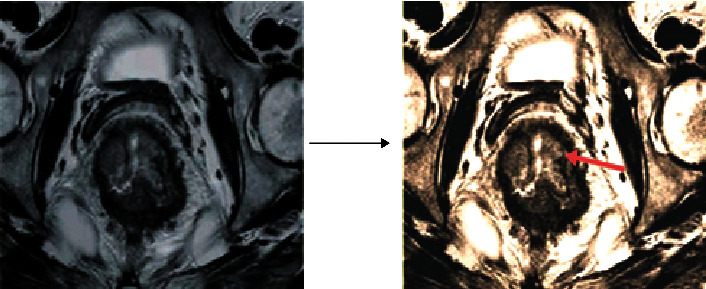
MRI images before and after low-rank matrix denoising processing (the red arrow indicated the lesion).
